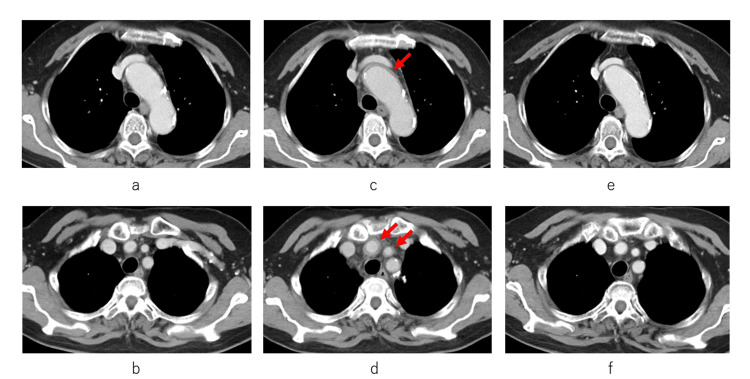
Serial contrast-enhanced computed tomography (CT) images showing changes in the aortic arch and supra-aortic vessels. (a, c, e) Aortic arch; (b, d, f) Brachiocephalic and common carotid arteries. (a, b) Baseline CT before the initial administration of filgrastim. (c, d) CT performed 8 days after initial filgrastim administration, depicting wall thickening consistent with vasculitis (arrows). (e, f) CT obtained 2 months after the onset of vasculitis, demonstrating an improvement in vascular inflammation.
